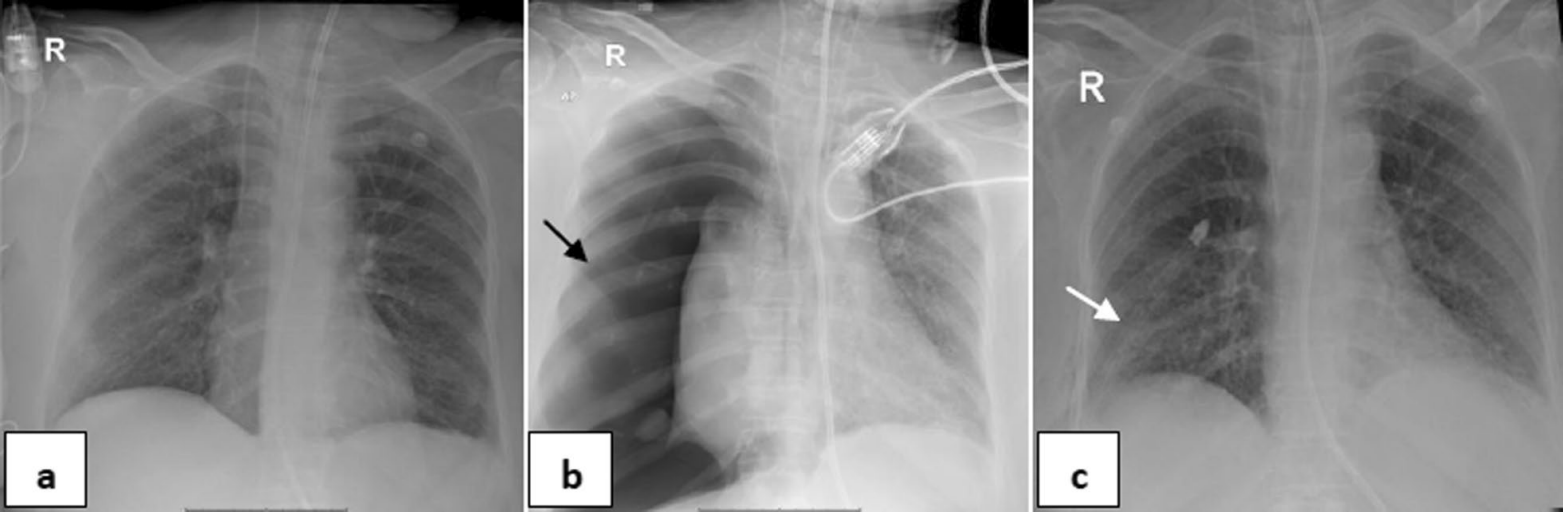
46-year-old male with no previous medical history, intubated 14 days post admission. a–c series frontal chest x-ray radiographs demonstrate a large right pneumothorax 4 days after intubation (black arrow). There is a right pleural pigtail catheter (white arrow) and re-expansion of the right lung. Note hazy interstitial densities throughout his lungs
